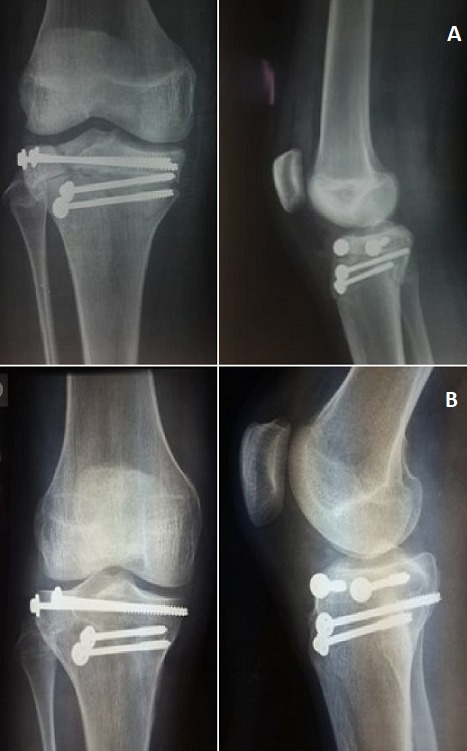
Radiographies post-opératoires: (A) post opératoire immédiat; (B) après un an de suivi
